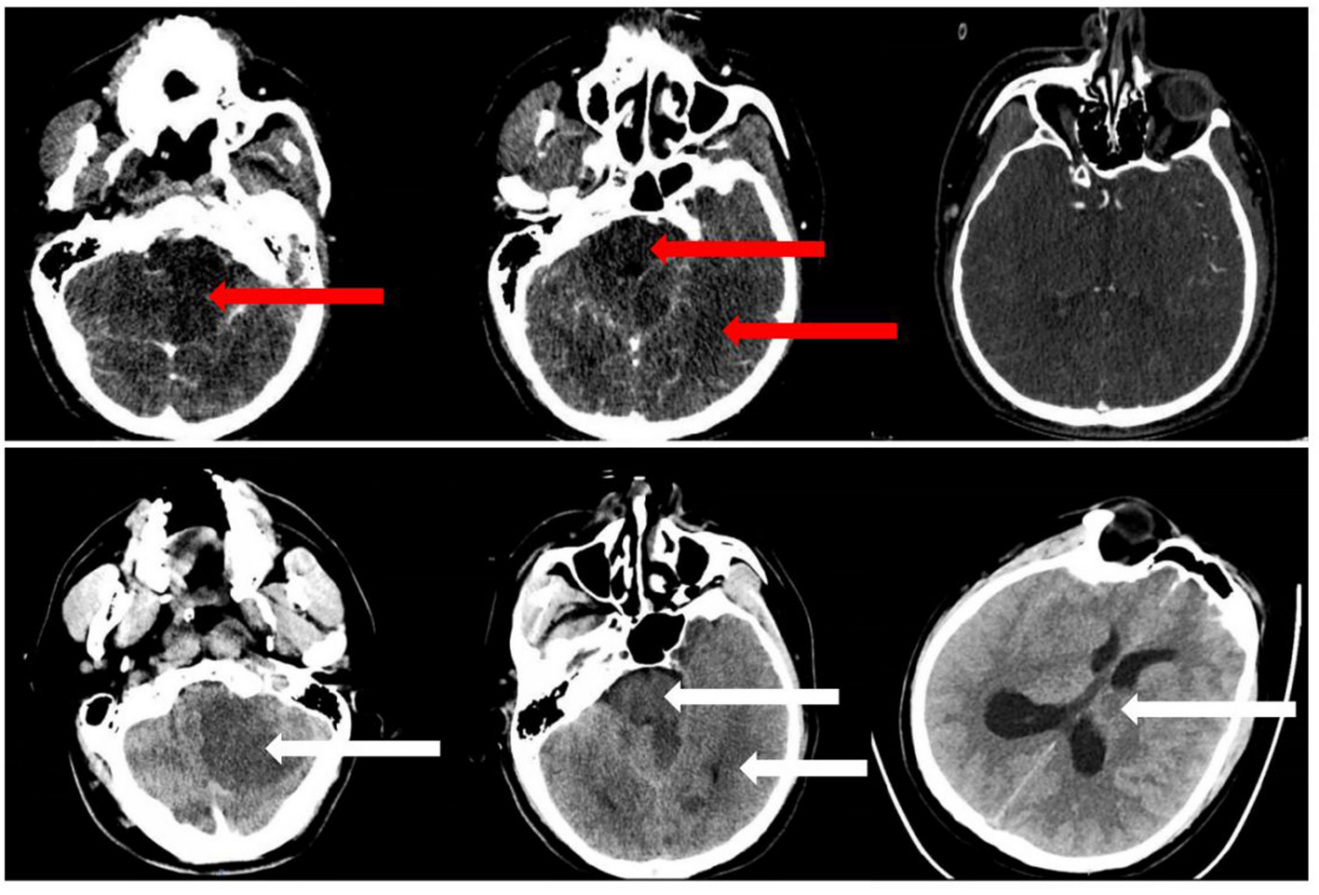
Example of a patient with BAO in our study. We rated CT angiography (CTA) source images (CTA-SI) (upper row) posterior circulation Acute Stroke Prognosis Early CT Score (pc-ASPECTS) score 6 (hypoattenuation left PCA territory, left cerebellum, midbrain) and follow-up non-contrast CT (NCCT) (lower row) pc-ASPECTS score 5 (additional ischemic change left thalamus).
